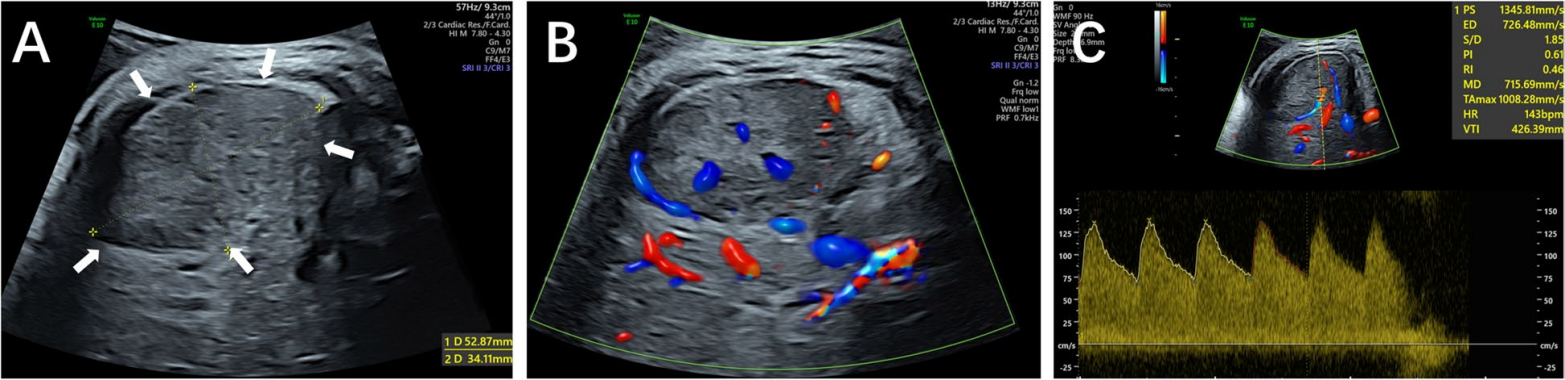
Prenatal ultrasonography imaging showed a voluminous, solid and heterogeneous hepatic mass. A Two-dimensional ultrasonography revealed a well-circumscribed, hypoechoic, and predominantly solid mass of approximately 5.3 × 4.0 × 3.4 cm in the right lobe of the fetal liver (arrow). B Color doppler showed a hypoechoic mass in the right liver of the fetus with abundant strip blood and peripheral blood. C Pulse doppler showed that the blood flow signal of the fetal right lobe mass displayed an arterial spectrum with a peak systolic velocity of the feeding artery of 1.35 m/s and a resistance index of 0.46
